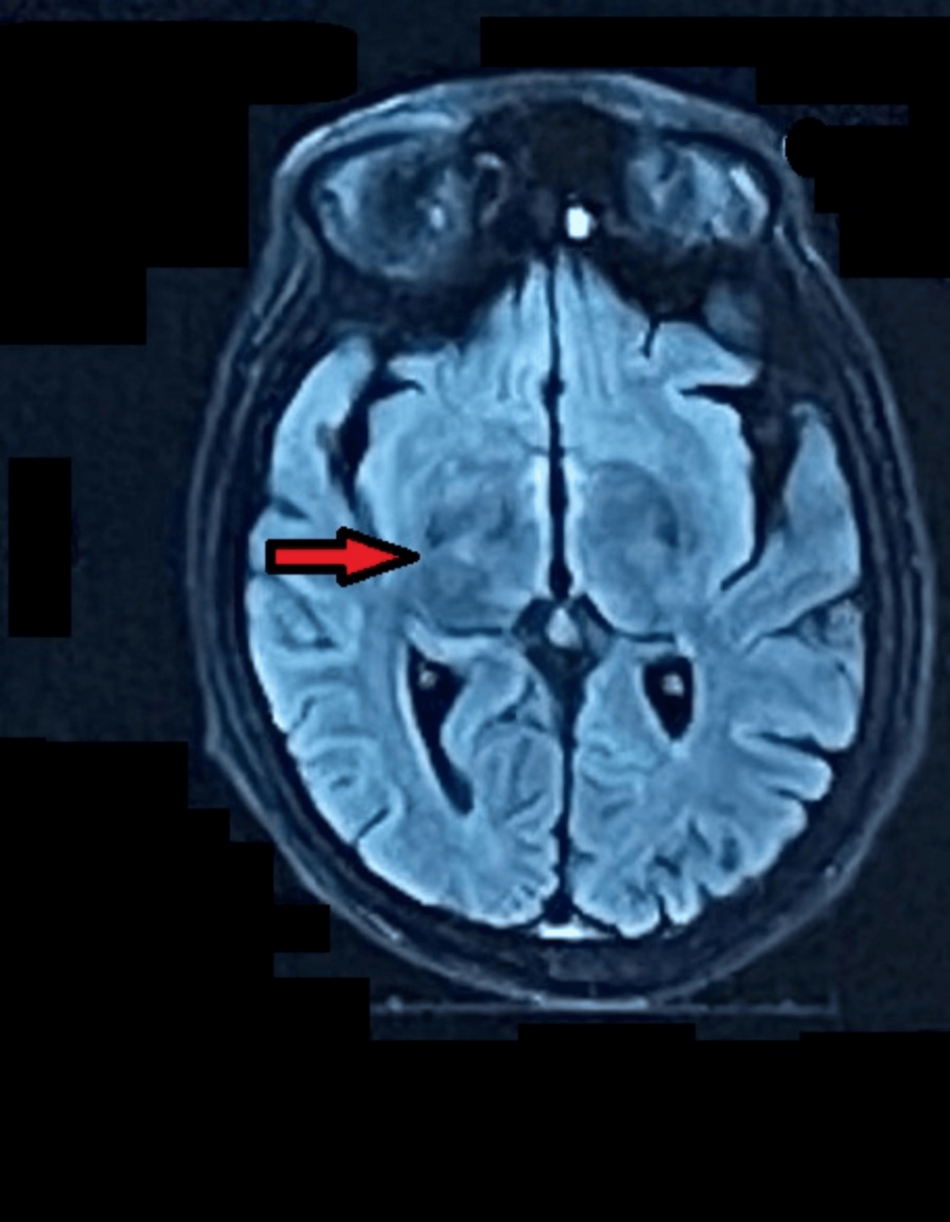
T1-weighted image of an MRI brain with contrast showing iron deposits in the basal ganglia, with patient identifying information digitally concealed in the image. Magnetic resonance imaging (MRI) of the brain with contrast showing hyperintense signals in the region of the basal ganglia, secondary to iron deposits, indicated by the red arrow. The image shows normal grey and white matter distribution. The ventricular system and extraventricular, CSF spaces appear to be normal.
